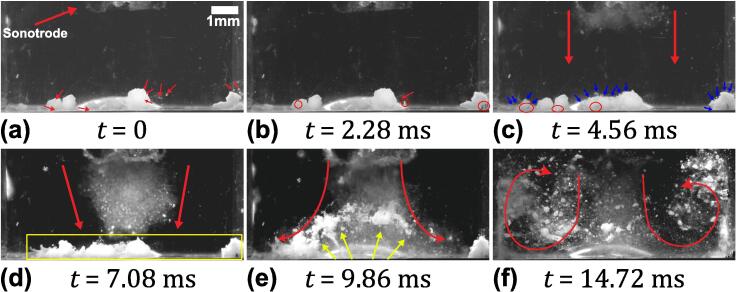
Sequence of images showing de-agglomeration and dispersion of MgO aggregates under the influence of ultrasound captured at 50 kfps with sonotrode amplitude of 210 μm peak-to-peak amplitude. See Supplementary video 4.
